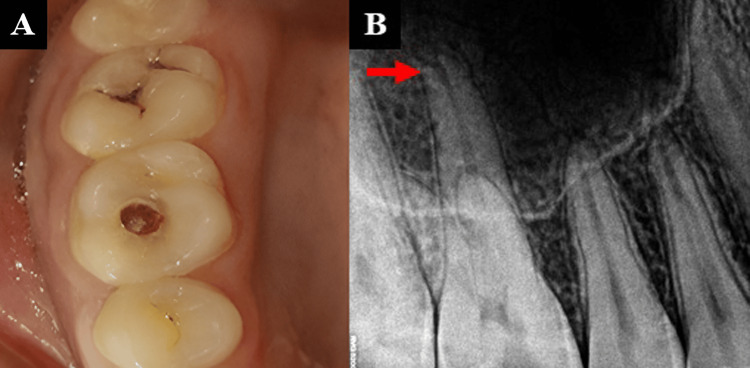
(A) Pre-operative clinical image and (B) pre-operative radiograph revealing an open apex.
